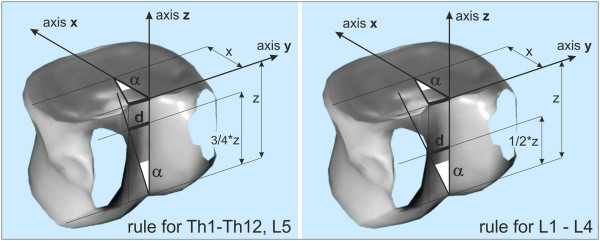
Principles for rules of radiographic evaluation of axial vertebral rotation. There are two bit different rules for thoracic spine and vertebra L5 (x = 0.75*z) and for lumbar spine L1 to L4 (x = 0.5*z).
